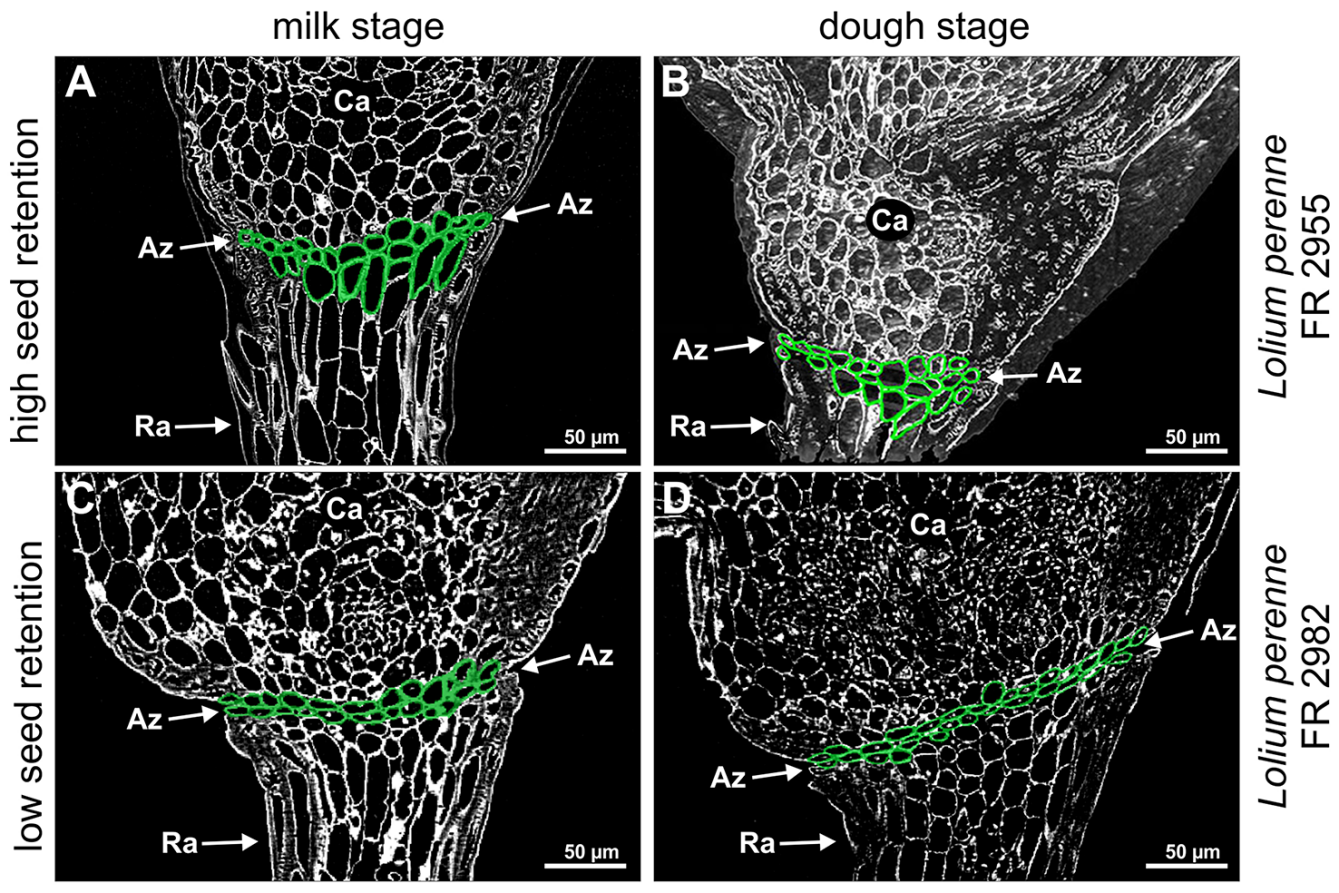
X-Ray analysis of the abscission zone of *Lolium perenne* at milk and dough stage. X-Ray analysis of tissue from milk (A, C) and dough stage (B, D) of accessions FR 2955 with high seed retention (A-B) and FR 2982 with low seed retention (C-D). Green cells indicate the abscission layer in the transition area between the rachilla and the developing caryopsis. Az, abscission zone; Ca, caryopsis; Ra, rachilla
